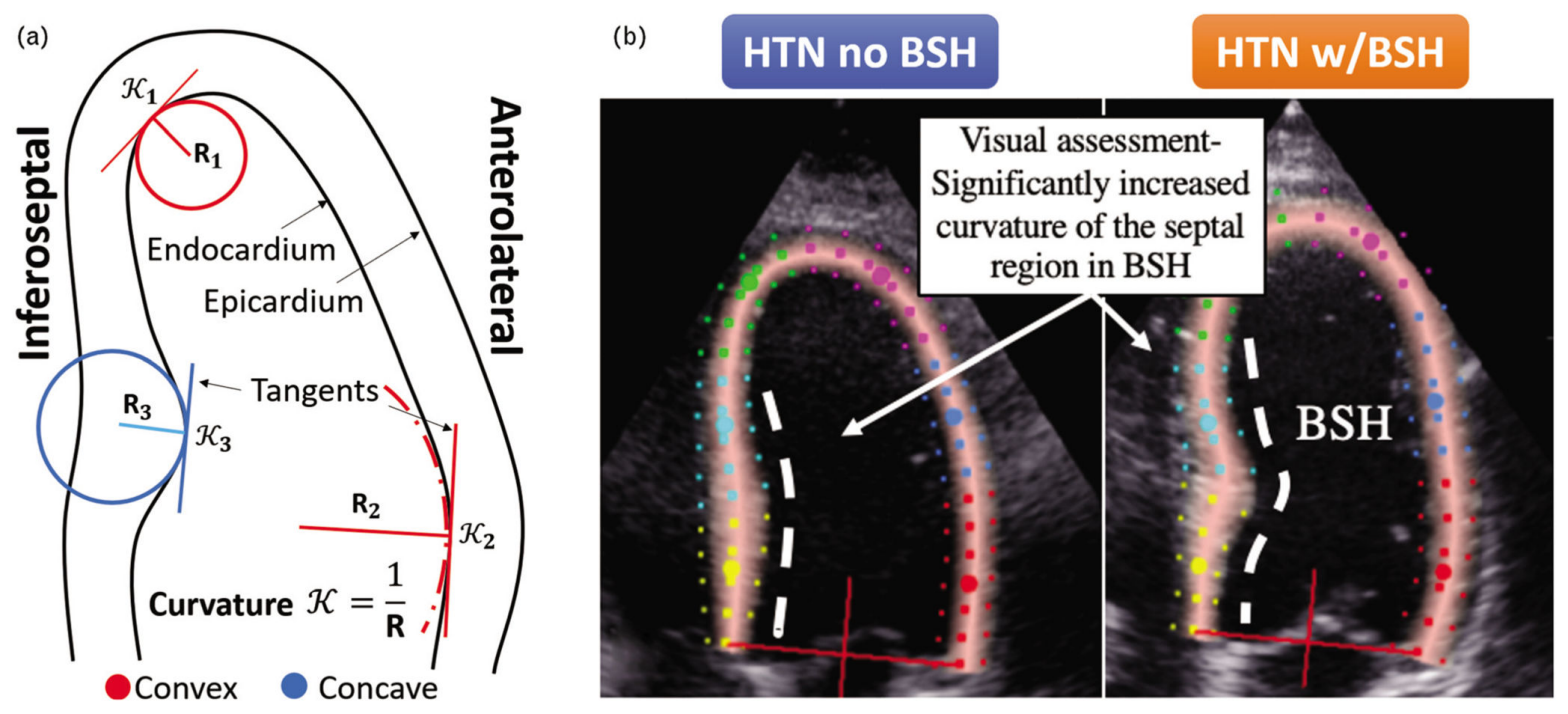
(a) An intuitive explanation of convex and concave curvature of the left ventricular endocardium, as seen in the four-chamber echocardiography view. The curvature is a reciprocal of the radius of circle adjacent to the endocardium. (b) The comparison of the septal wall in hypertensive patients with and without the basal septal hypertrophy (BSH). The visual assessment differentiating the two can be quantitatively compared with the curvature metric.
